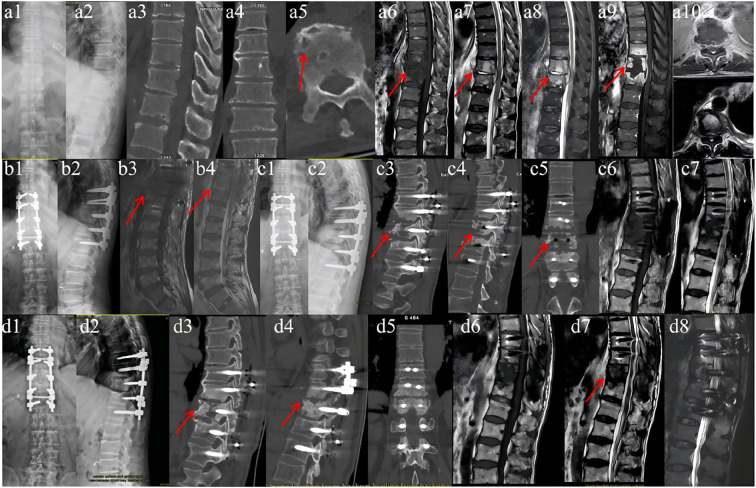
Preoperative and postoperative follow-up imaging of a patient with T9–T11 *Escherichia coli* infection treated with direct pedicle screw fixation involving the infected vertebrae. Preoperative imaging: (a1, a2) Anteroposterior and lateral radiographs. (a3–a5) Sagittal and axial CT images revealing micronodular bone destruction lesions (arrows). (a6–a10) Sagittal and axial MRI demonstrating bone marrow edema in the T9–T11 vertebral bodies, as well as abscesses in the intervertebral spaces and spinal canal, with severe destruction of the T10 vertebral body (arrows). Postoperative imaging at 1 month: (b1, b2) Anteroposterior and lateral radiographs. (b3, b4) Sagittal MRI indicating abscess clearance with no obvious recurrence (arrows). Postoperative imaging at 3 months: (c1, c2) Anteroposterior and lateral radiographs. (c3–c5) Sagittal and axial CT images revealing resolution of edema, the actual extent of bone destruction after abscess disappearance, and internal fixation in good position without loosening (arrows). (c6, c7) Sagittal MRI demonstrating no recurrence of abscess (arrows). Postoperative imaging at 12 months: (d1, d2) Anteroposterior and lateral radiographs. (d3–d5) Sagittal CT images demonstrating newly formed sclerotic bone in the lesion area, with internal fixation in good position and no fracture detected (arrows). (d6–d8) Sagittal MRI confirming that the patient achieved good recovery with no recurrence of abscess (arrows).
